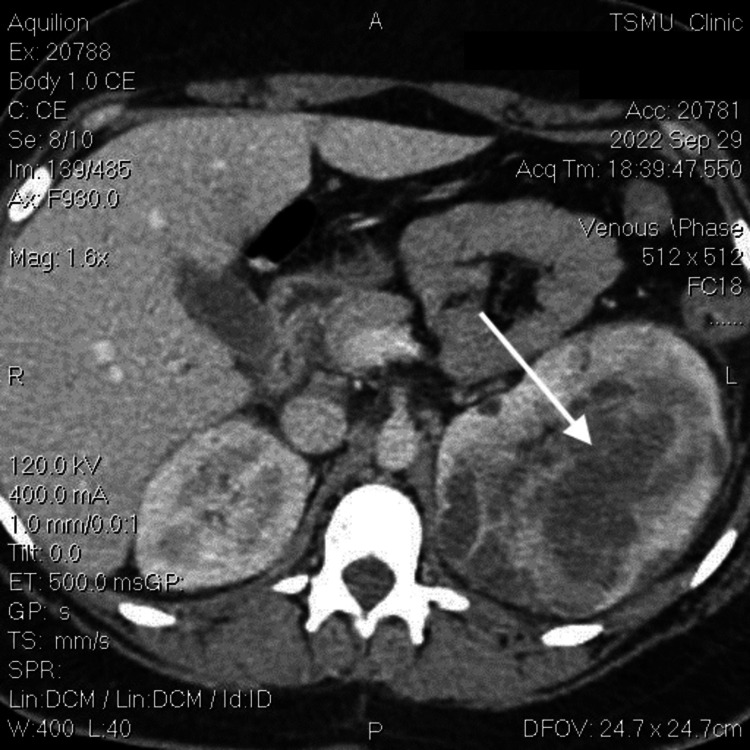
Axial view of CT scan showing left-sided loculated renal abscess (white arrow). CT: computed tomography.
